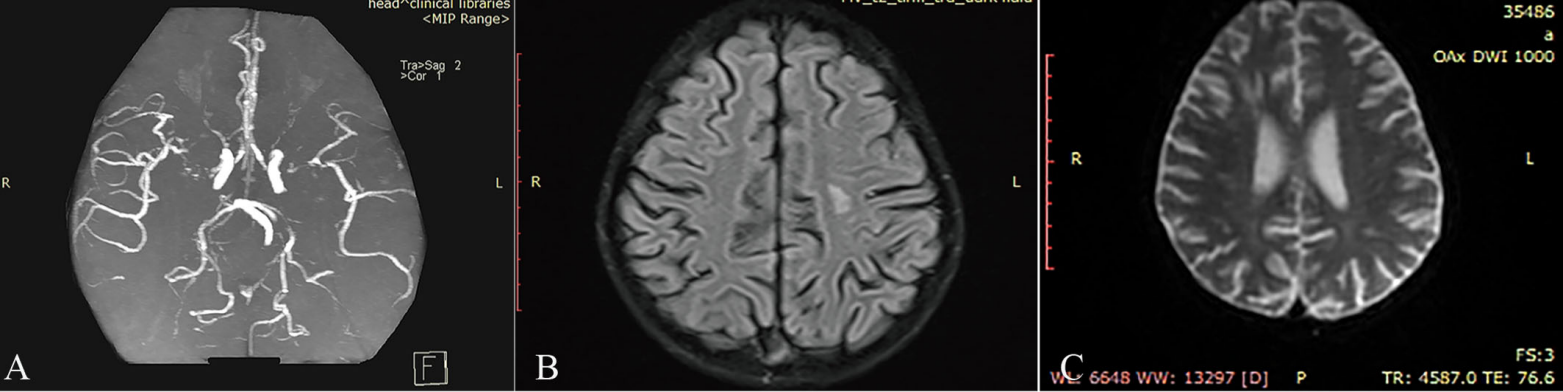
(A) Pre-chemotherapy brain MRA: segmental interruptions of bilateral internal carotid artery (ICA) siphon segments and middle cerebral arteries (MCA), with severe stenosis/occlusion and multiple tortuous collateral vessels. (B) Brain MRI: new high signal in the left semioval center on T2-weighted sequences, suggestive of ischemic lesion or demyelination. (C) Brain MRI DWI: new acute ischemic lesions in the right basal ganglia, indicating recent cerebral infarction.
